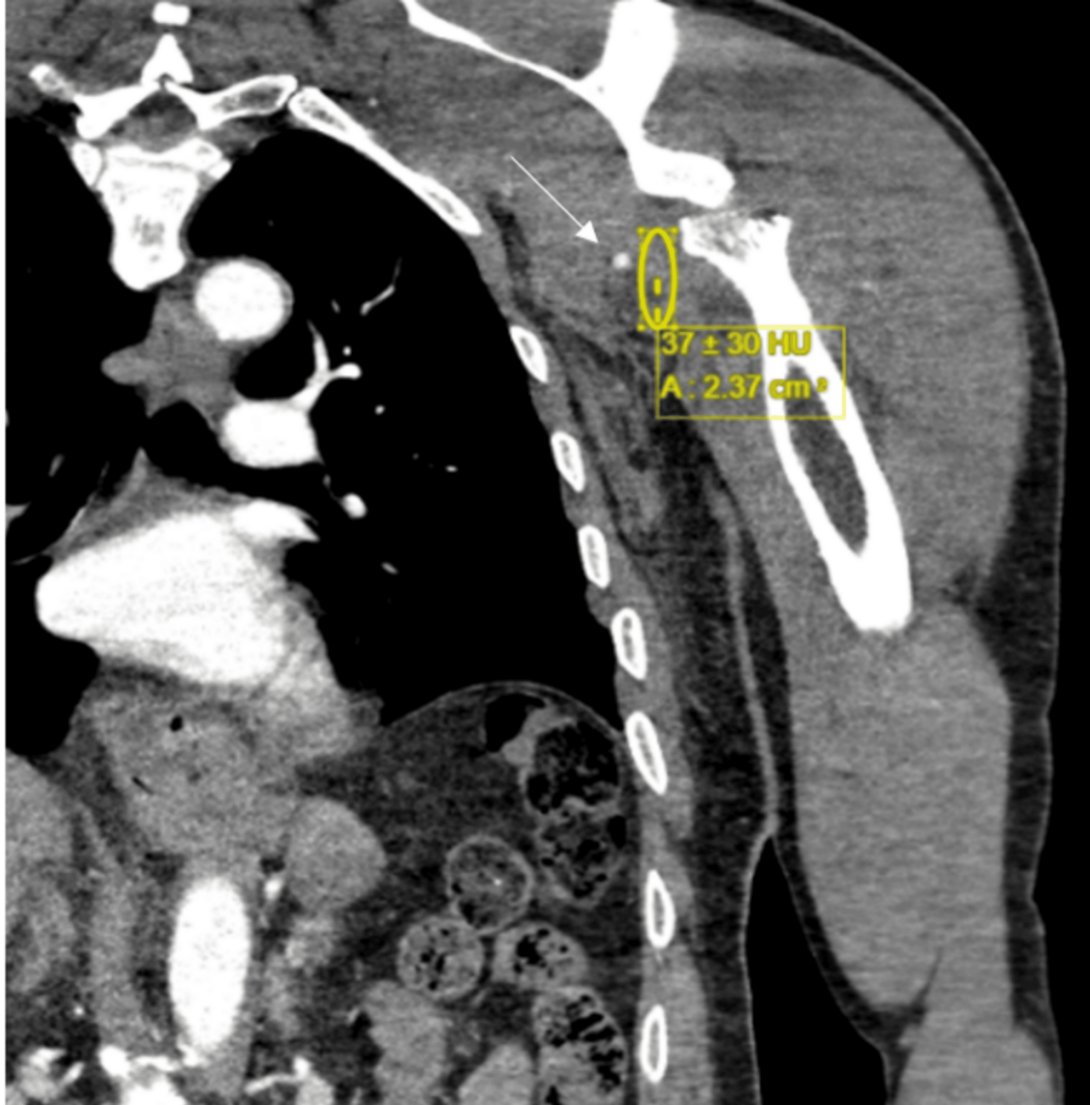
Coronal CTA of the left upper limb demonstrating axillary artery transection The white arrow indicates the position of the axillary artery. CTA: computed tomography angiogram
